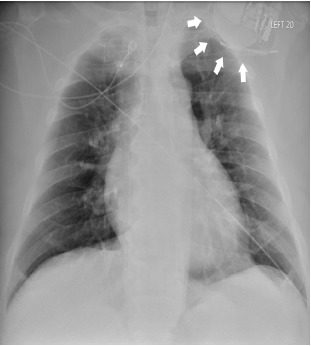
Anteroposterior chest radiograph revealing automatic implantable cardioverter-defibrillator leads wrapped around the device (white arrows) and absence of leads in the ventricle.
